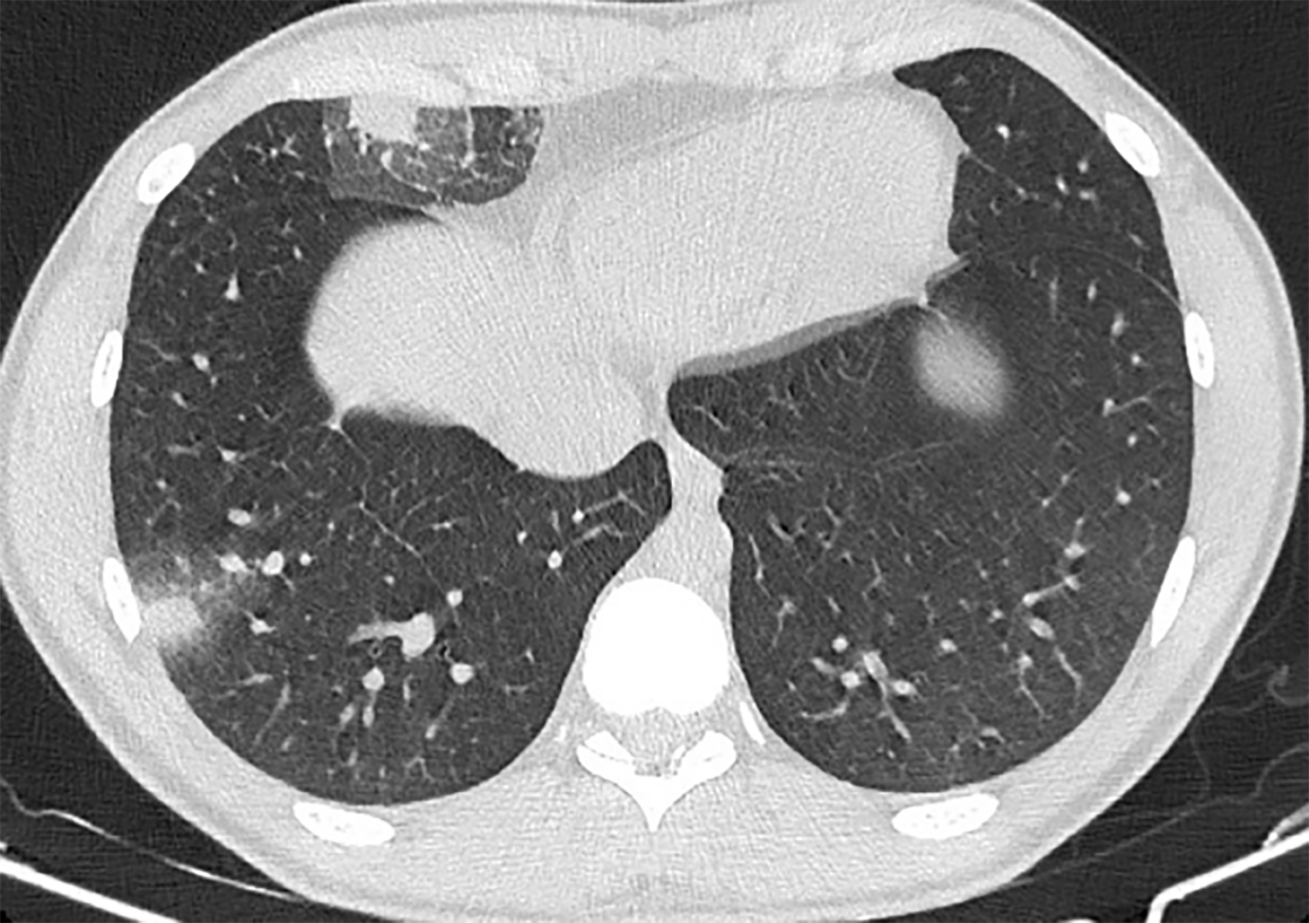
A 24-year-old man with a history of Crohn’s disease, currently on infliximab and azathioprine, presented with a two-week history of cough, fever, chills, night sweats, and dyspnea after visiting a cave. A chest CT revealed two nodules with slightly irregular contours and a ground-glass halo, located in the peripheral region of the right lower and middle lobes. He was diagnosed with probable pulmonary histoplasmosis after positive serology.
